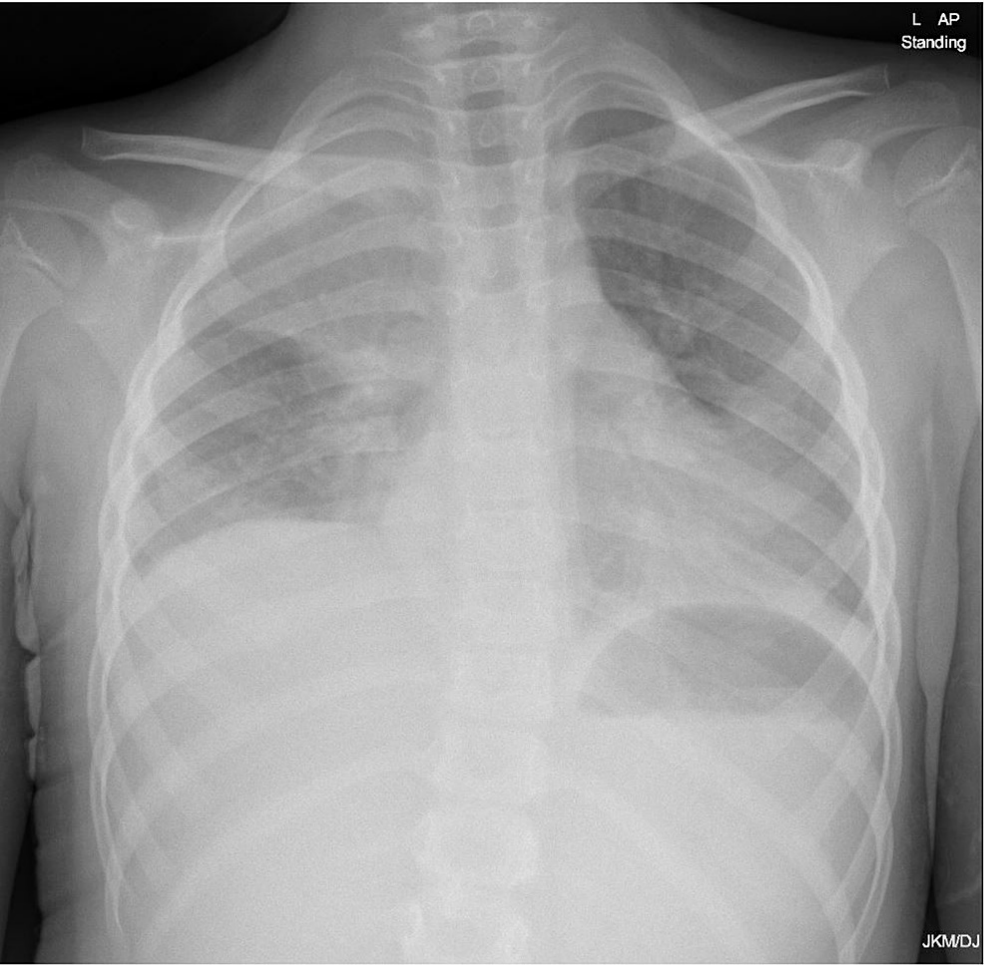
Repeat CXR showing new consolidation on the left side of the lung. CXR: chest X-ray
